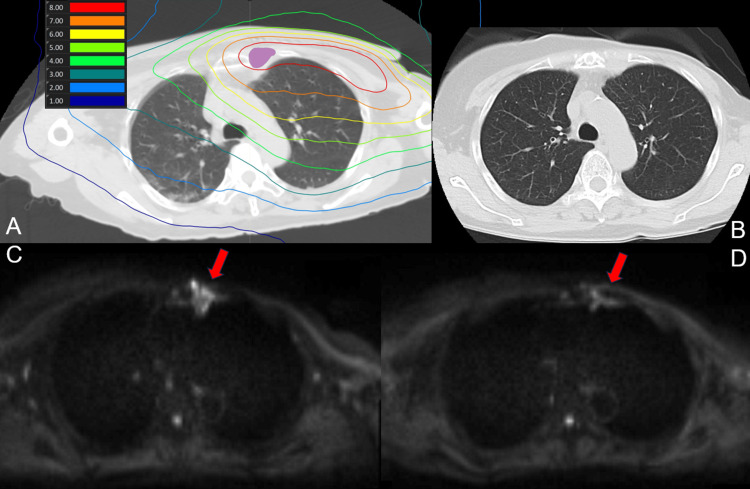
(A) Dose distribution map for BNCT (lung). Purple represents the GTV. (B) CT scan 90 days post-treatment shows no signs of radiation pneumonitis. (C) Pre-treatment diffusion-weighted imaging MRI. (D) MRI scan 90 days shows a decrease in signal intensity.
